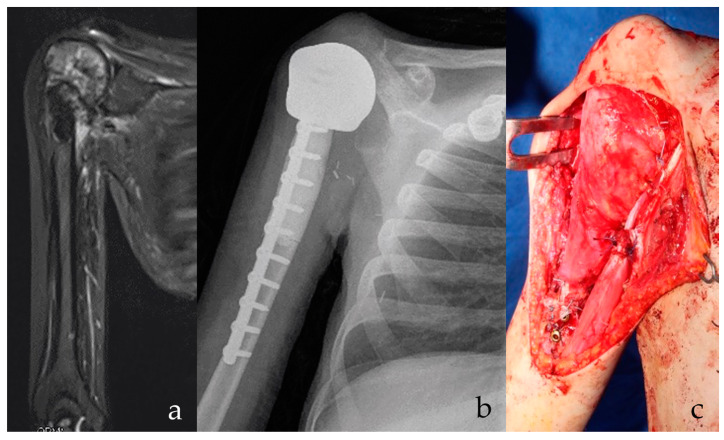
(a) Osteosarcoma of the proximal humerus in Li Fraumeni Syndrome is shown on radiography. Possible infected biopsy tract. Patient had prior brain medulloepithelioma with residual parethic lower limb. Stable and effective shoulder function are essential for everyday activities. (b) After intraarticular proximal humerus and deltoid resection, a custom-made, 3D-printed anatomical proximal humerus reconstruction with diaphyseal antibiotic cement is implanted. At 15 months follow-up, the implant is stable. (c) A latissimus dorsi rotational flap provides for functional recovery of the deltoid and offers a biological barrier to infection. A stable and effective implant is shown, with low risks of secondary infection.
